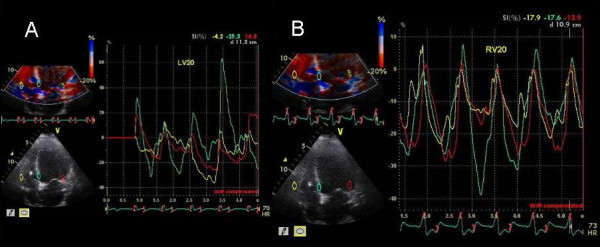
Strain maps in the apical 4 chamber view at LV pre-excitation of 20 ms (A) and right ventricular pre-excitation of 20 ms (B). Note improvements in strain velocities from an average of 15 cm/s during LV pre-excitation to an average of 20 cm/s during right ventricular pre-excitation. In addition improved synchrony is also seen in the basal LV and right ventricular segments. Yellow line represent basal right ventricle, blue represents basal inferior interventricular septum and red represents basal lateral segment.
